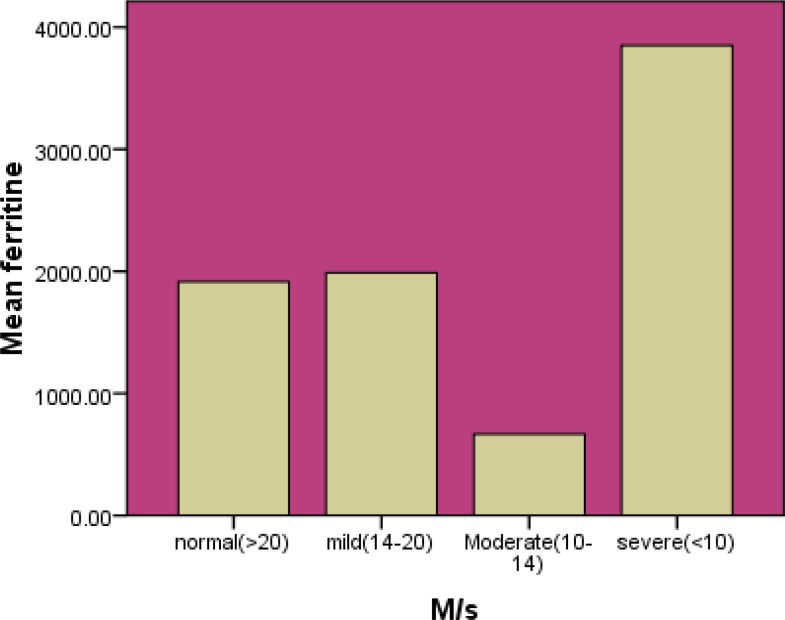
Serum levels of ferritin and cardiac T2*MRI
